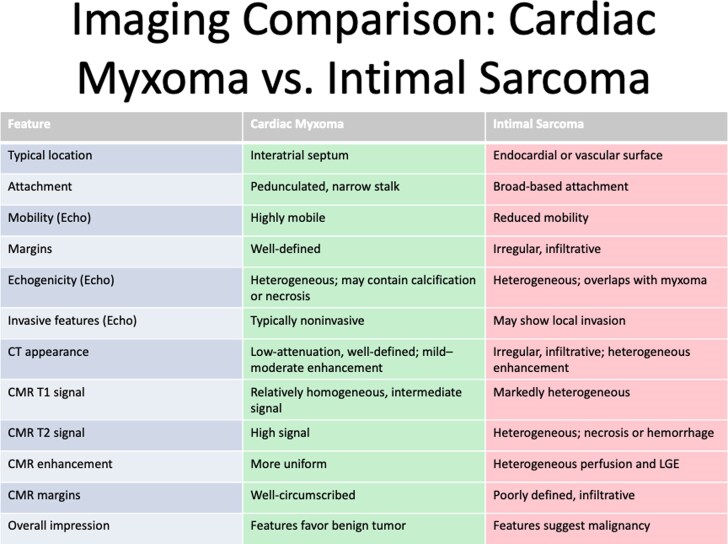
Imaging comparison of Echo, CT, and MRI of myxoma vs. sarcoma.
